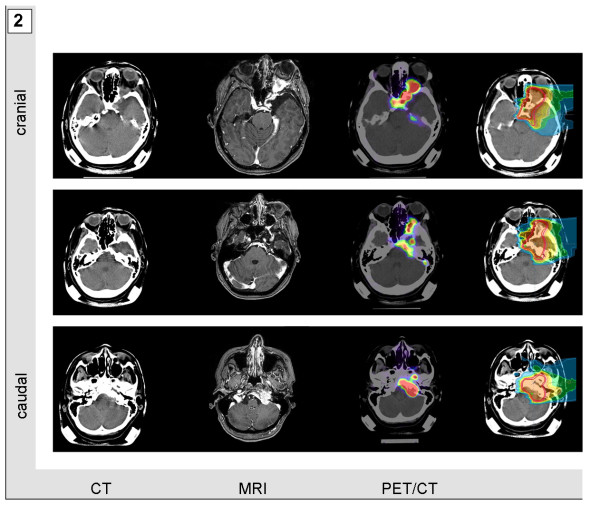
Multifocal diffusely spreading atypical meningioma in a 55-year-old woman. Contrast-agent enhanced CT and MRI scan were fused with a DOTATOC-PET/CT examination and used to calculate a two beam carbon ion radiotherapy plan.
